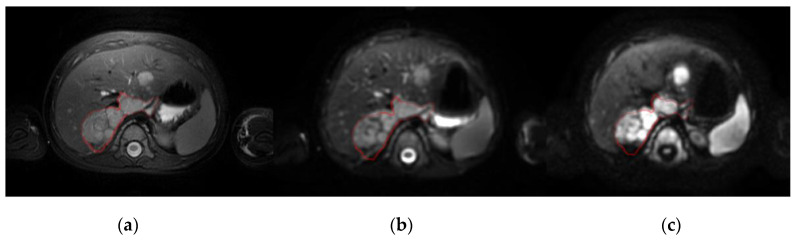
Baseline MRI of a patient with neuroblastoma in the right adrenal gland and liver metastasis shown on: (a), a T2-weighted image; (b), a DW image with b = 0 s/mm^2^; and (c), a DW image with b = 800 s/mm^2^ (right). The red line defines the primary tumor.
